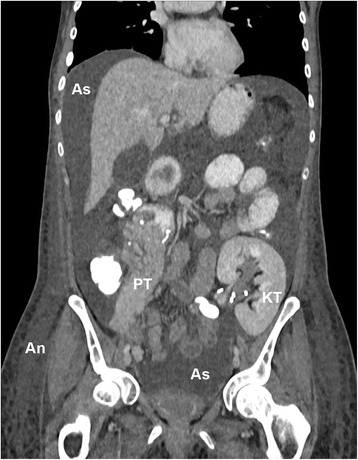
CT-scan in the parenchymal phase after iv-injection of iodinated contrast agent on day 18 after combined kidney-pancreas transplantation. Small bowel is filled with oral contrast-agent, colon structures with rectal contrast agent. The kidney transplant (KT) in the left lower abdomen still exhibits a ureteral stent. In the upper part of the pancreas transplant (PT) the anastomosis to the small bowel is visible. Both, kidney and pancreas grafts display normal contrast agent uptake behavior. Extensive ascites (As) can be seen in the four quadrants of the abdomen, moreover anasarca (An) is shown in the subcutaneous tissue of both flanks
